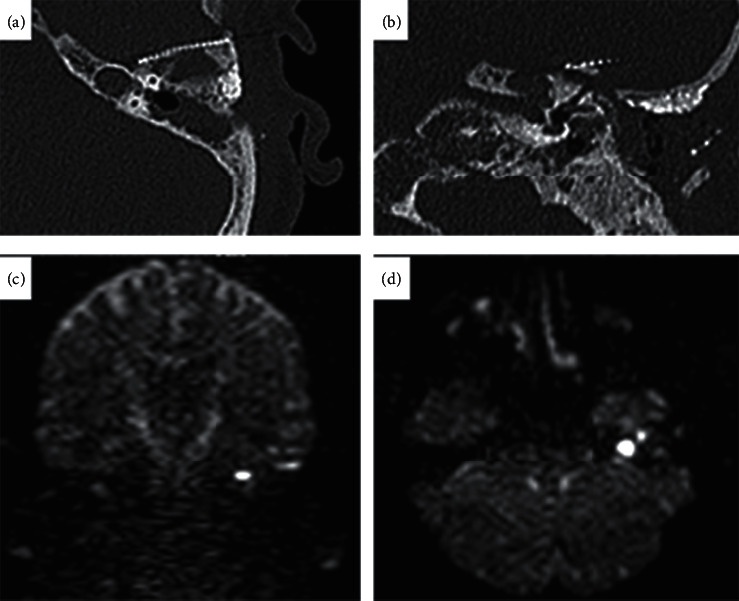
(a, b) Preoperative axial CT showing (asterixs) the petrous apex with bone erosion and a titanium plate covering the partially covering anterior limitation of the temporal bone. (c, d) Non-EPI DWI with coronal and axial planes. Note the diffusion restriction in the petrous apex of the left temporal bone.
